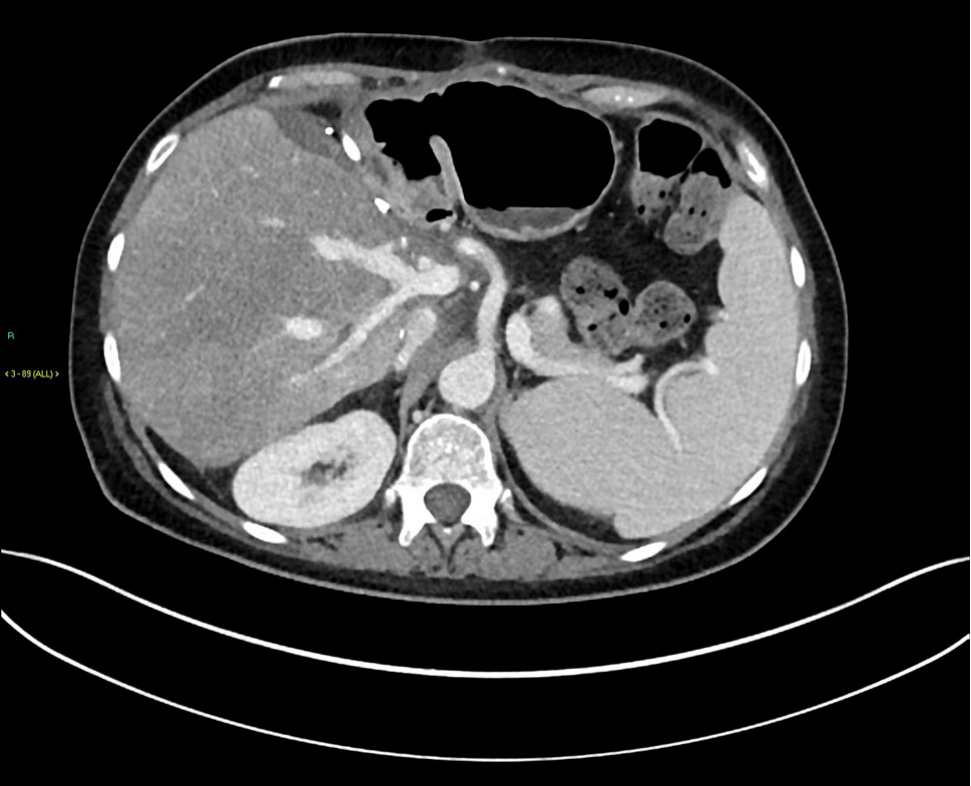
Axial contrast-enhanced CT post-extended left hepatectomy with resection and reconstruction of IVC
